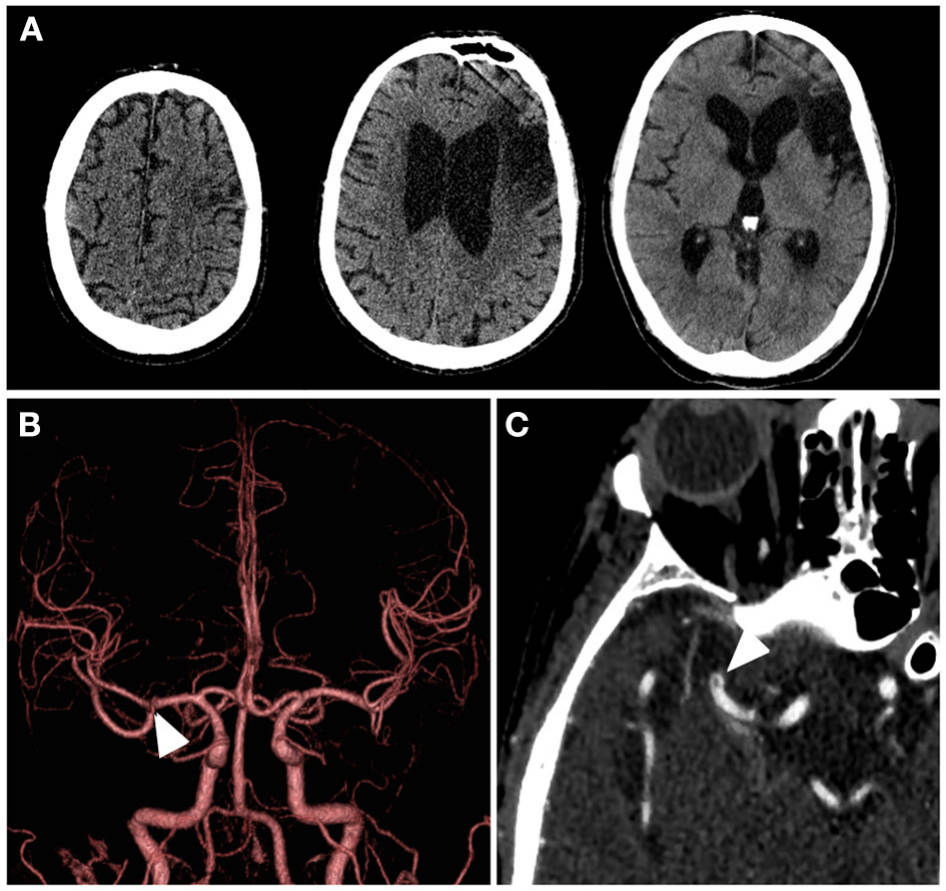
Patient's cerebral imaging at the time of symptom onset. (A) Computed tomography showed an obsolete infarction of the left frontal lobe, but there were no findings suggestive of hemorrhage or acute infarction on the diseased side. (B) Computed tomography angiography with three-dimensional volume rendering did not exhibit obvious filling defects, but a localized microstenosis was noted in the right middle cerebral artery distal M1 segment (white arrowhead). (C) Subocclusive thrombus in the right middle cerebral artery distal M1 segment (white arrowhead) was found on close observation through the axial image of the computed tomography angiography with maximum intensity projection.
